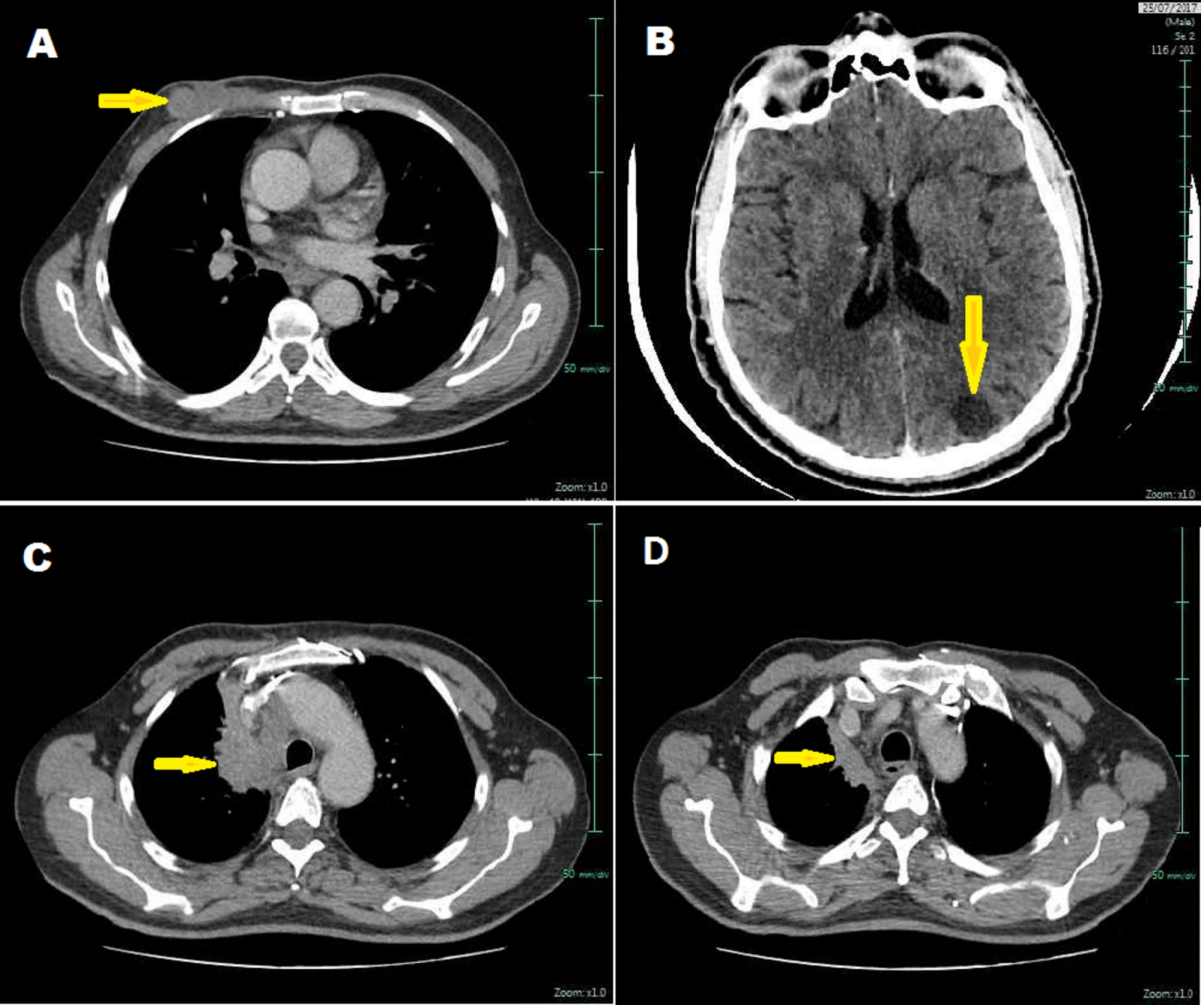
Cerebral, cervicothoracic, and abdominal computed tomography (CT) scans A) right breast mass, B) brain metastasis, C-D) right apical lung tumor
